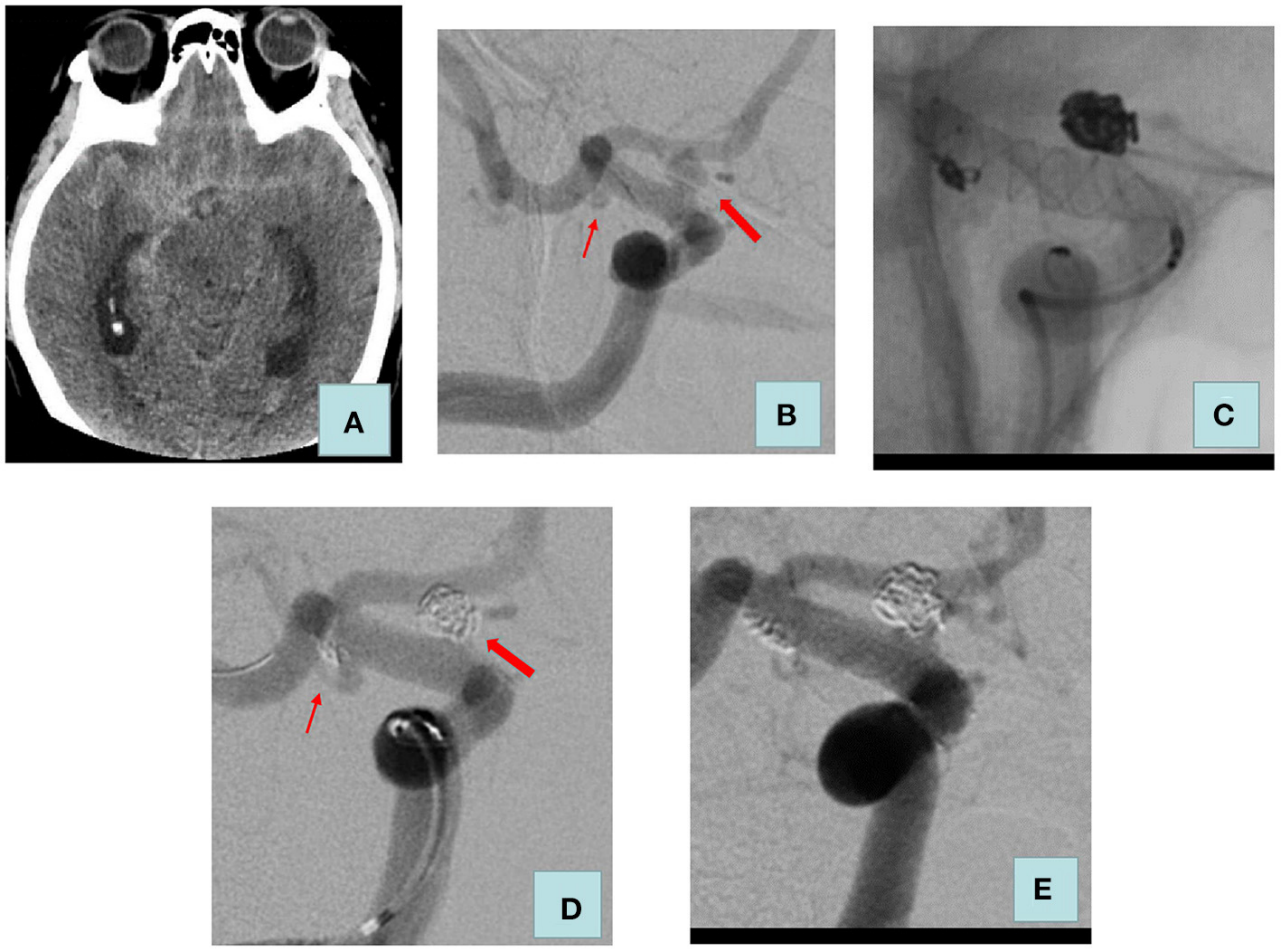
Adult patient presented with severe headache for 5 h. (A) Computed tomography of the head revealed a diffuse subarachnoid hemorrhage. (B) Cerebral angiography showed a blood blister aneurysm (size: 3.9 * 3.5 mm) and a saccular aneurysm (size: 2.1 * 1.0 mm) of the right internal carotid artery C7 segment. (C) We used LVIS stent-assisted coils embolizing the two aneurysms. (D) Raymond–Roy classification immediately was as follows: ruptured aneurysm, complete embolization, unruptured aneurysm, and sac remnant. (E) Review 4 months after surgery: the two aneurysms were complete embolization. The thick arrow indicates a ruptured aneurysm; the thin arrow indicates an unruptured aneurysm.
